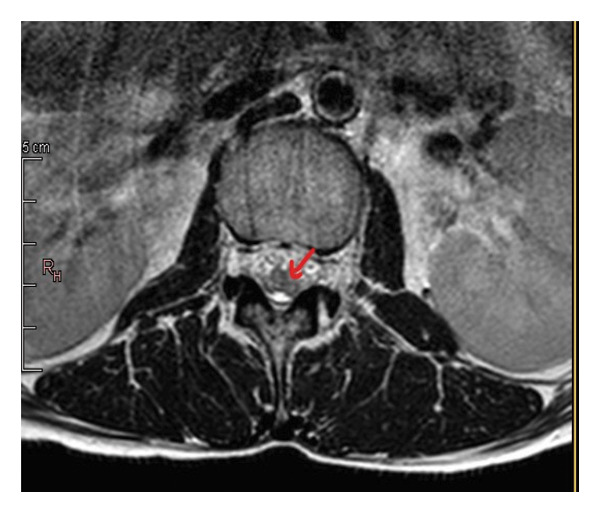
Spinal MRI, axial section with T2 weighting, showing a hypersignal occupying more than 50% of the spinal cord.
